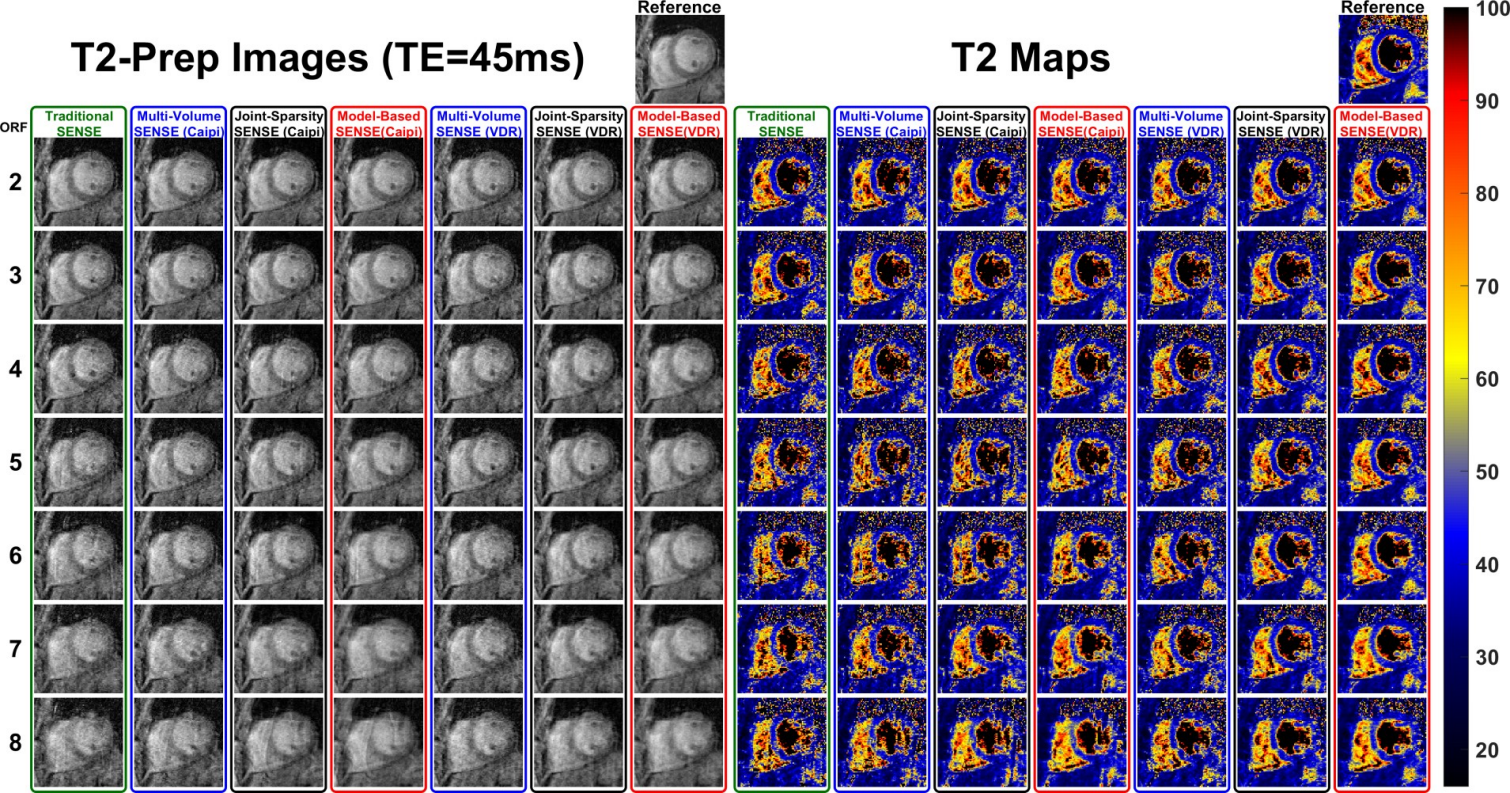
T2-weighted images and T2 maps of a normal human subject with ORF = 2–8 and all reconstruction approaches. T2-Prep TE is 45 ms in the T2-weighted images. As ORF increases (top to bottom), images from all approaches appear noisier and blurrier, and additionally images from traditional SENSE and Caipi sampling patterns suffer from ghosting artifacts. The complete dataset including all slices for all reconstructions can be seen in S1 Video.
